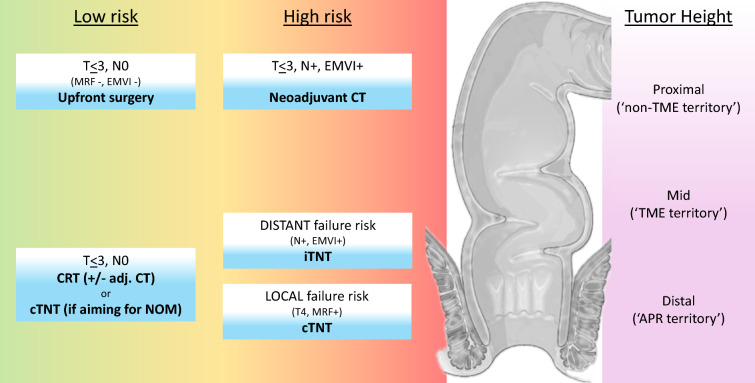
Summary of neoadjuvant therapy options in LARC adj., adjuvant; APR, abdominoperineal resection; CRT, chemoradiotherapy; CT, chemotherapy; c, consolidation; EMVI, extramural venous invasion; i, induction; LARC, locally advanced rectal cancer; MRF, mesorectal fascia; mets., metastases; NOM, non-operative management; TME, total mesorectal excision; TNT, total neoadjuvant therapy
